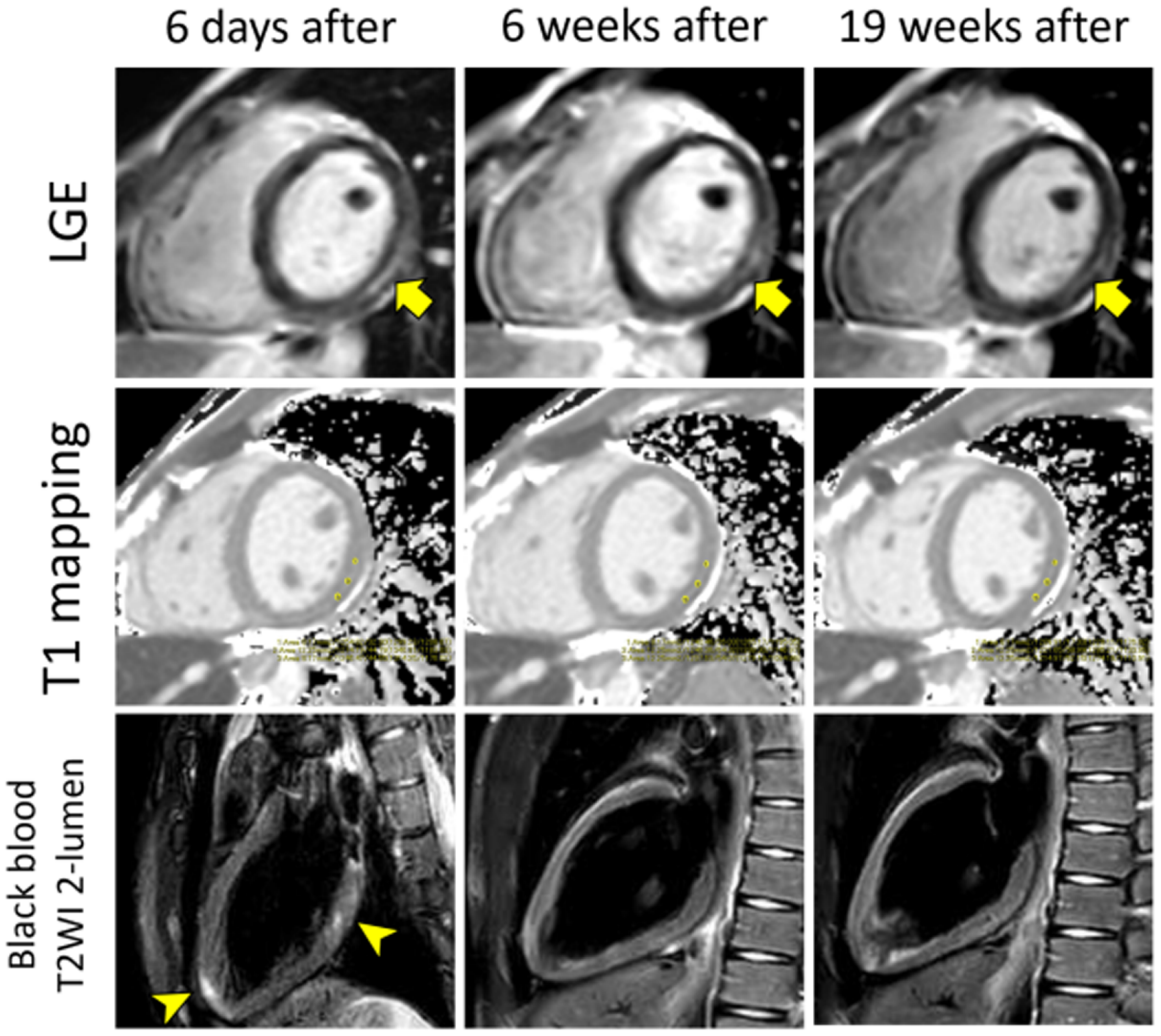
Cardiac MRI imaging at short axis from 6 days after onset (baseline), 6 weeks after onset (second MRI), and 19 weeks after onset (third MRI). Yellow arrows depict myocardial LGE. Native T1 values were within the normal range in the areas determined to be LGE positive. Black blood T2MI 2‐lumen showed areas of high signal intensity in the posterior wall and apex of the left ventricle on initial examination (yellow mark) but there were no abnormal findings on subsequent examinations. LGE, late gadolinium enhancement; MRI, magnetic resonance imaging.
